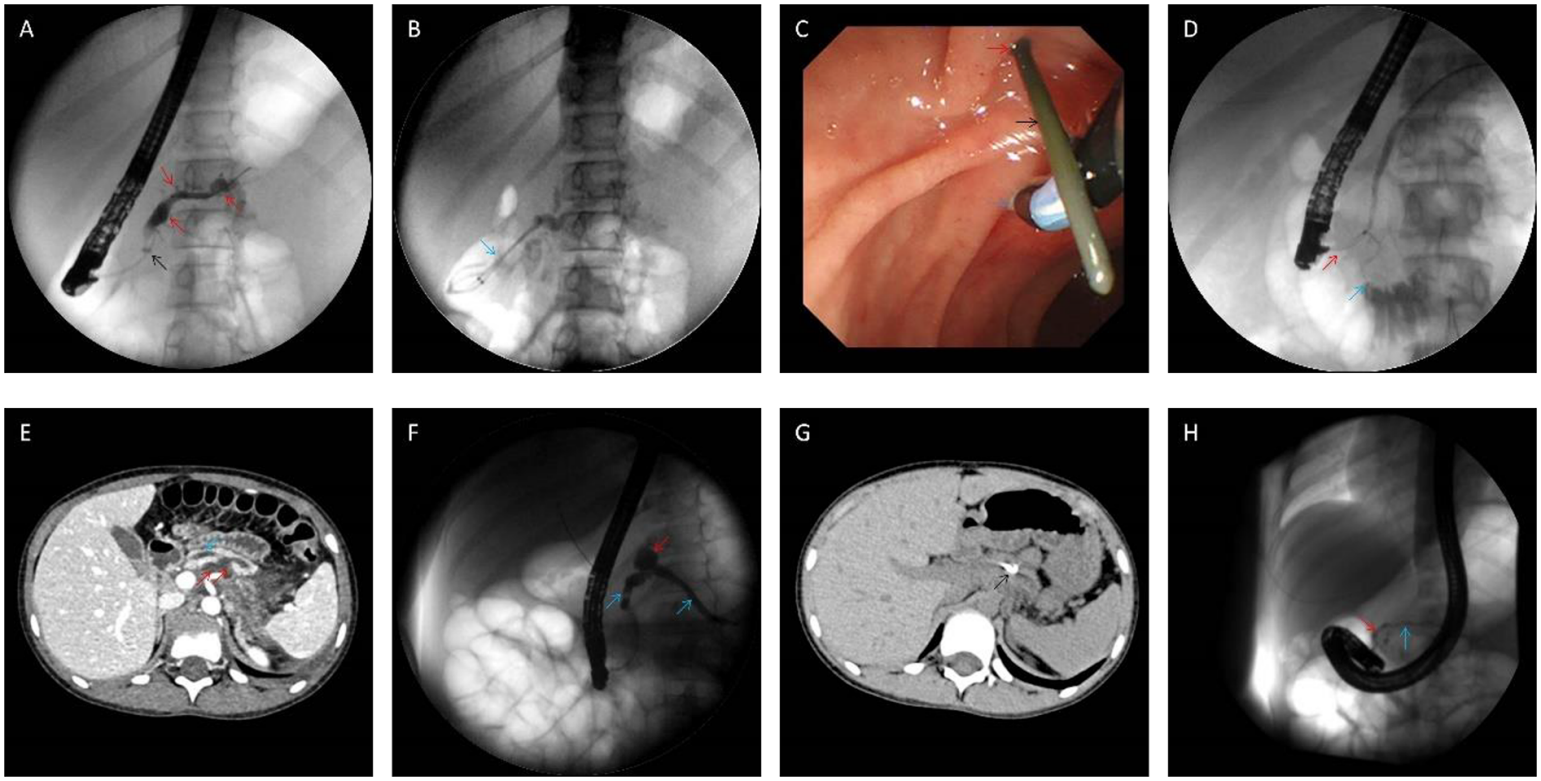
Intraoperative findings during endoscopic retrograde cholangiopancreatography. (A,B) Intraoperative findings of the fifth procedure in Case 2. The main pancreatic duct and branch pancreatic ducts were irregularly dilated (red arrow), and the proximal pancreatic duct was narrowed (black arrow); two pancreatic stents (5Fr-5 cm) (blue arrow) were placed for drainage after balloon dilatation of the stenosed area; (C,D) intraoperative image of the first ERP procedure in Case 6, she experienced spontaneous nipple intubation (the major papilla is marked with a blue arrow), which resulted in the penetration of the guide wire (black arrow) from the accessory nipple (red arrow), Incomplete PD confirmed by selective minor papilla intubation; (E–G) case 7 CT and pancreatography showed pancreatic duct dilatation (blue arrow), and the cyst (red arrow) communicate with the main pancreatic duct (E,F), reexamination at 2 months after operation showed that the stent (black arrow) was in place and the cyst was absorbed (G); (H) in Case 7, only the ventral pancreatic duct (red arrow) was visualized by the main papilla cannulation, and the accessory papilla cannulation showed the main pancreatic duct (blue arrow), but no communicating branches were found in the ventral and dorsal pancreatic ducts. Accordingly, the diagnosis of complete PD was made.
